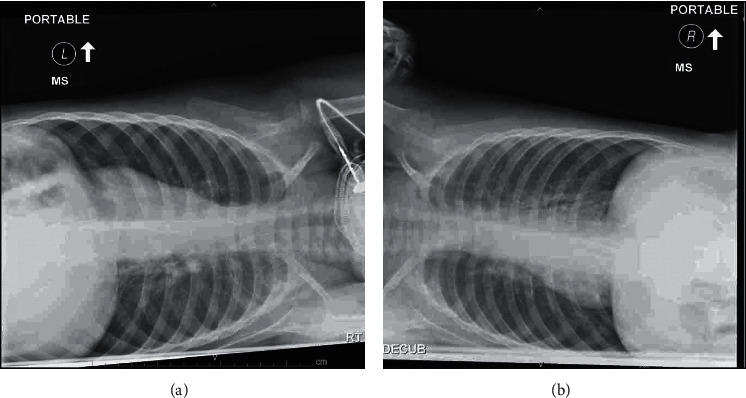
(a) Right and (b) left lateral decubitus chest X-rays taken after the EIT device was used in attempt to confirm diagnosis of FBA by more traditional means. The attending pediatric radiologist read these images: “Both lungs are hyperinflated on the decubitus view without significant asymmetry which suggests air trapping secondary to bronchiolitis.”
